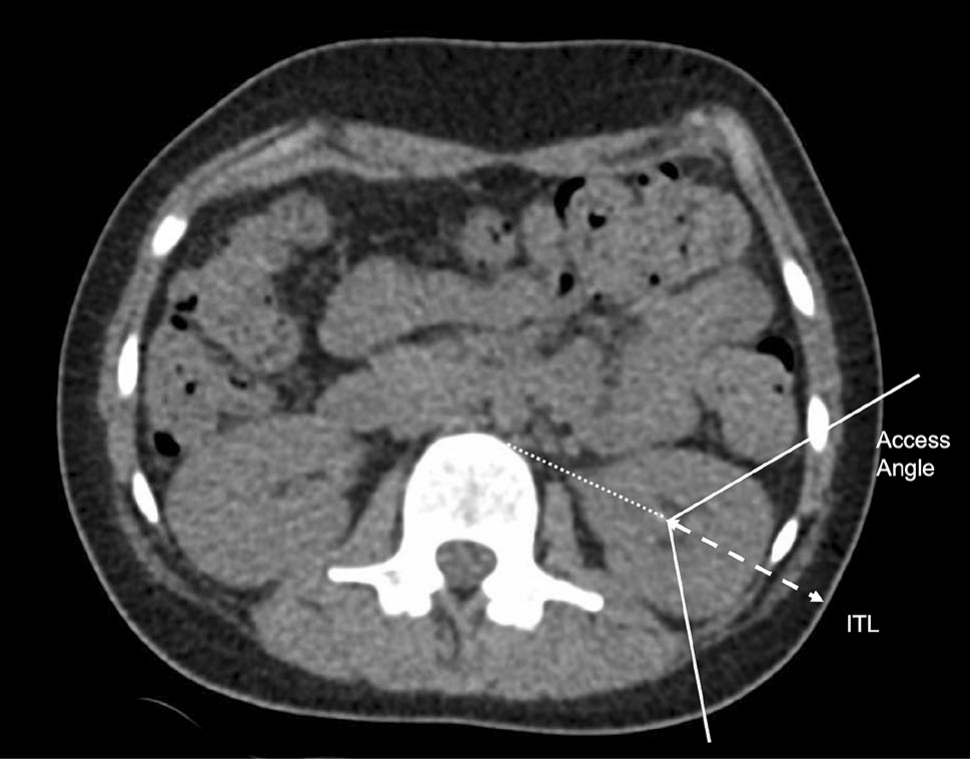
Ideal tract length (ITL) with the presence of ribs in the access angle measured on axial CT images
